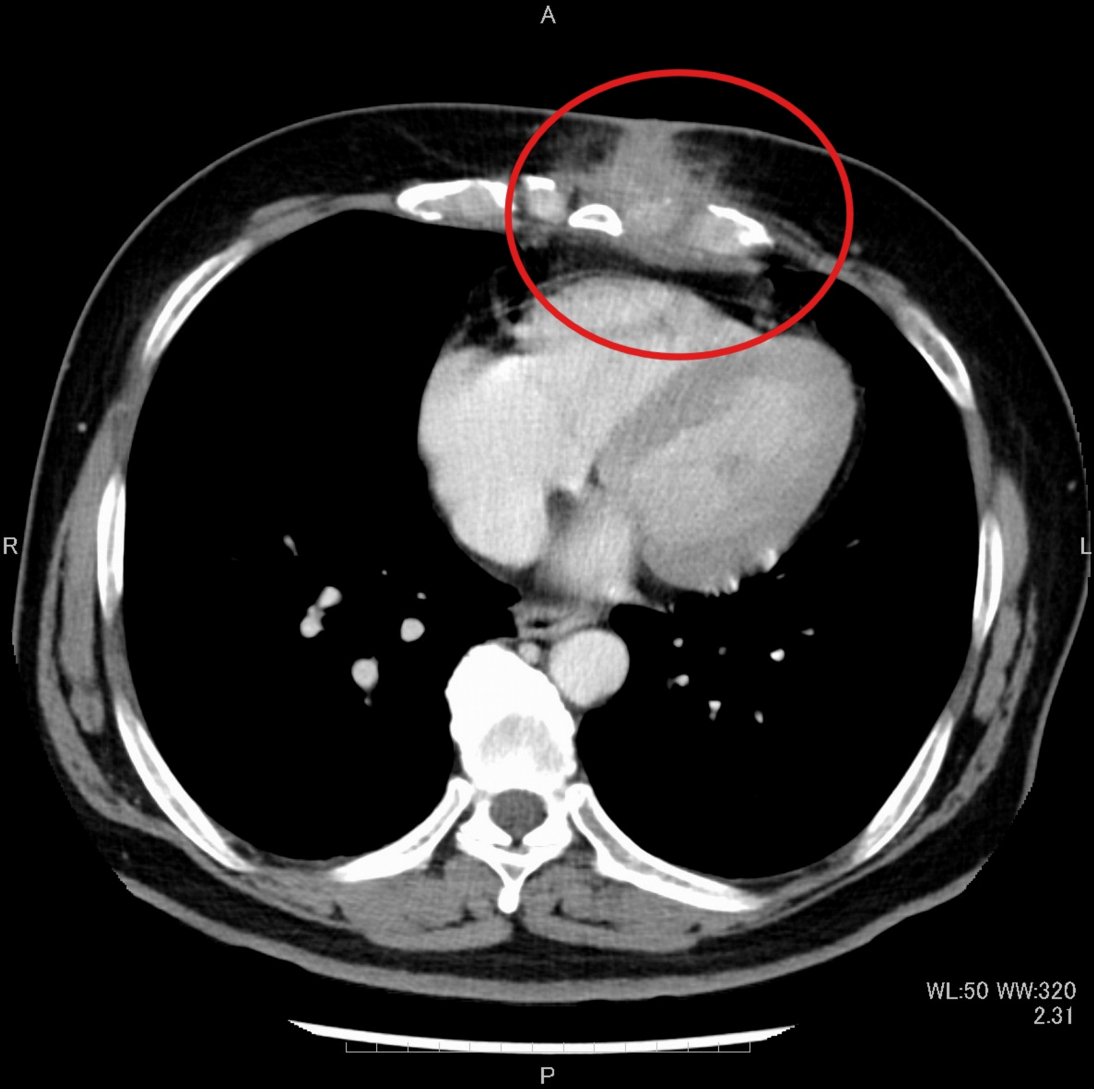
Computed tomography showing a high-density area around the inferior end of the sternum and surrounding subcutaneous tissue. The seventh costal cartilage is distracted (red circle)
